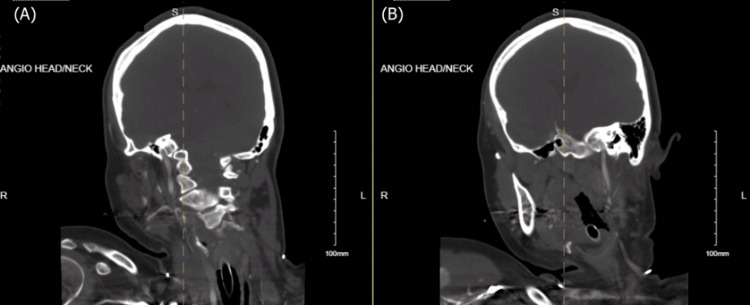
CTA head and neck from outside the hospital demonstrating lack of intracranial contrast with flow beyond the skull base at the (A) right and (B) left internal carotid arteries CTA, computed tomography angiography
